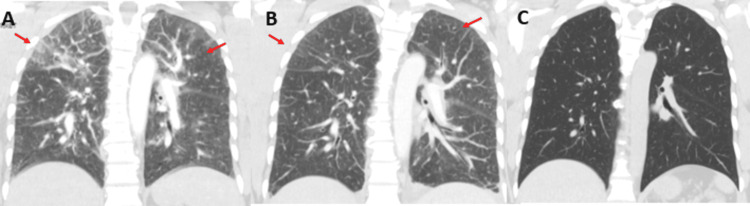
Changes in lung infiltration on CT scan 1A: CT pulmonary angiography. Diffuse bilateral ground-glass and interstitial opacities. Admission day. 1B: CT chest with contrast. Interval decrease in bilateral patchy ground-glass opacities. Admission day 4. 1C: CT chest without contrast. Interval resolution of patchy ground-glass opacities. Twelve days after discharge.
